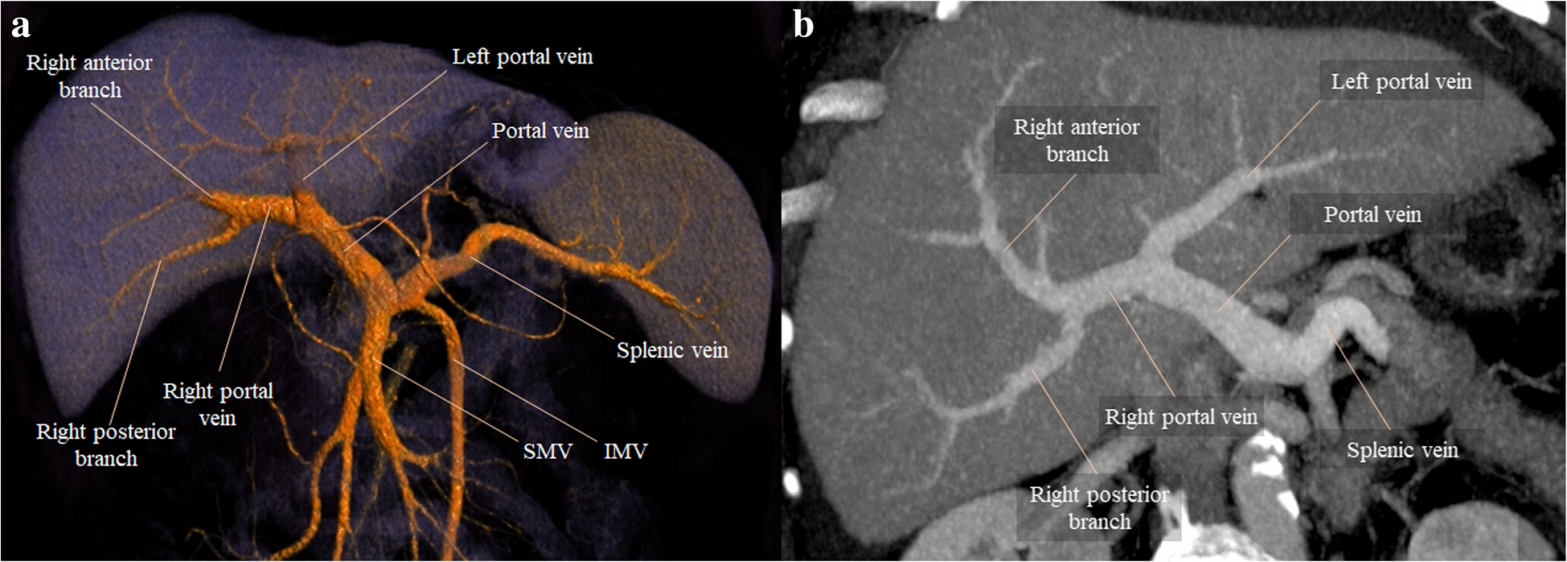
Normal anatomy of the PVS and the typical branching pattern of the main PV. a Volume rendering technique (VRT) image from late arterial phase contrast-enhanced CT depicting a global view of the PVS. b Maximum intensity projection (MIP) image from portal venous phase contrast-enhanced CT showing the typical branching pattern of the main PV
